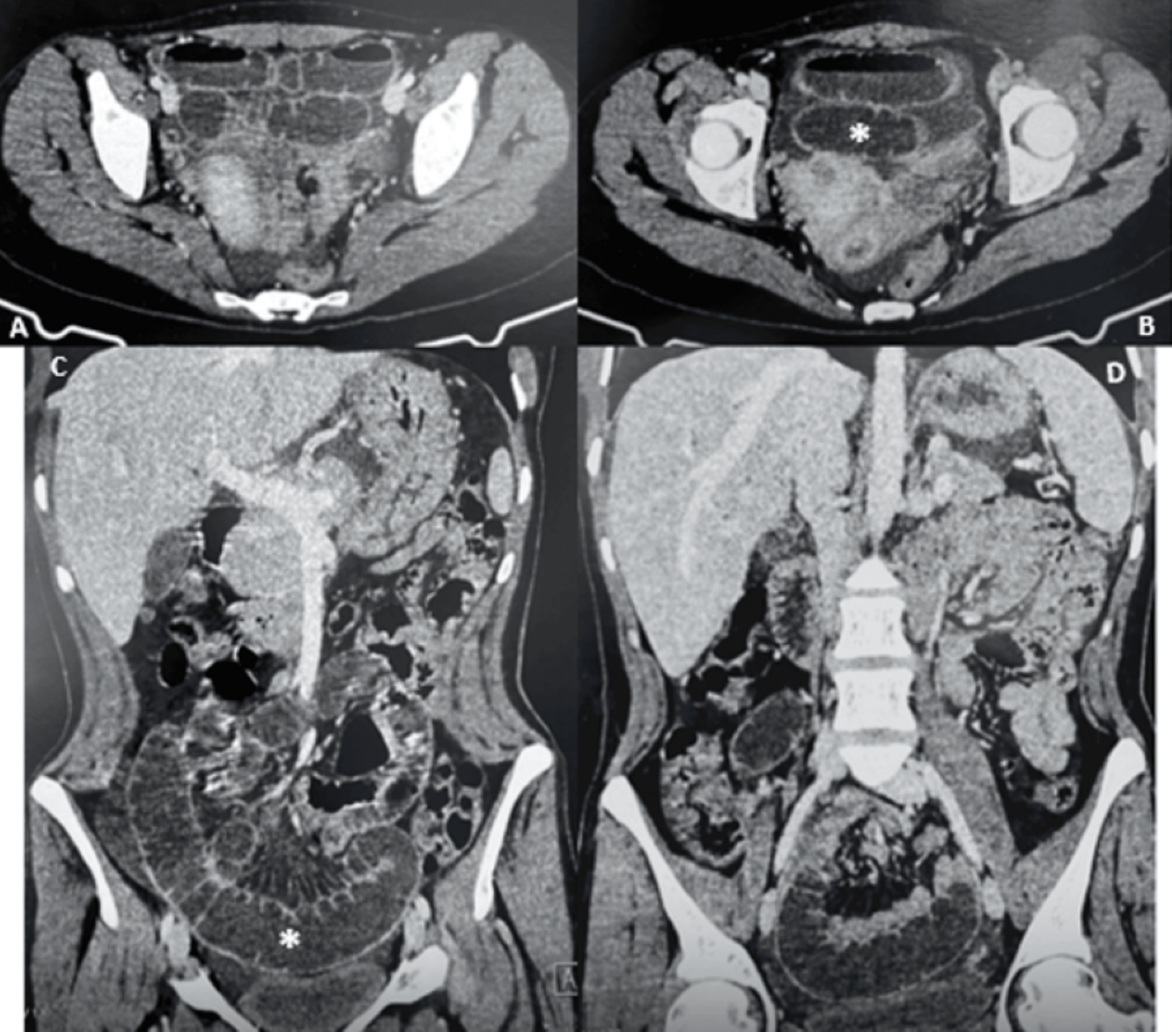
CT scan showing acute bowel obstruction, dilated ileum and air-fluid levels (*). (A) and (B) Axial view and (C) and (D) Coronal view
